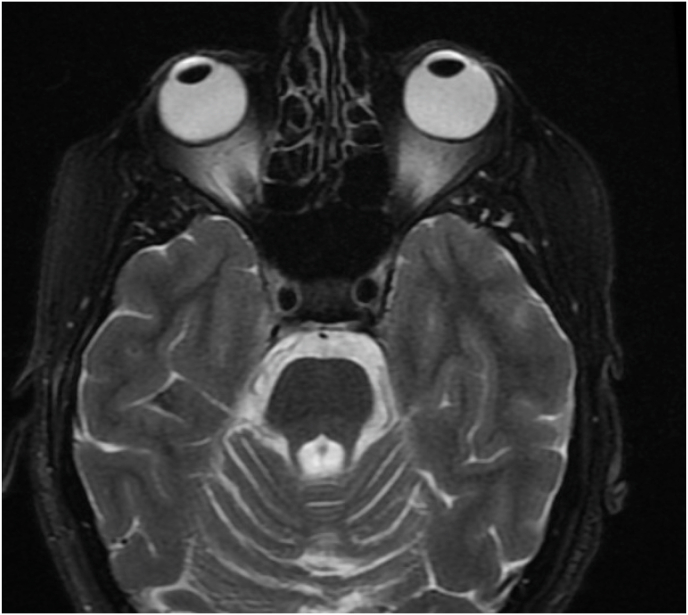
T2-weighted magnetic resonance image of displaying atrophy of the cerebellum and cerebellar peduncle bilaterally consistent with spinocerebellar degeneration in Machado-Joseph Disease.
